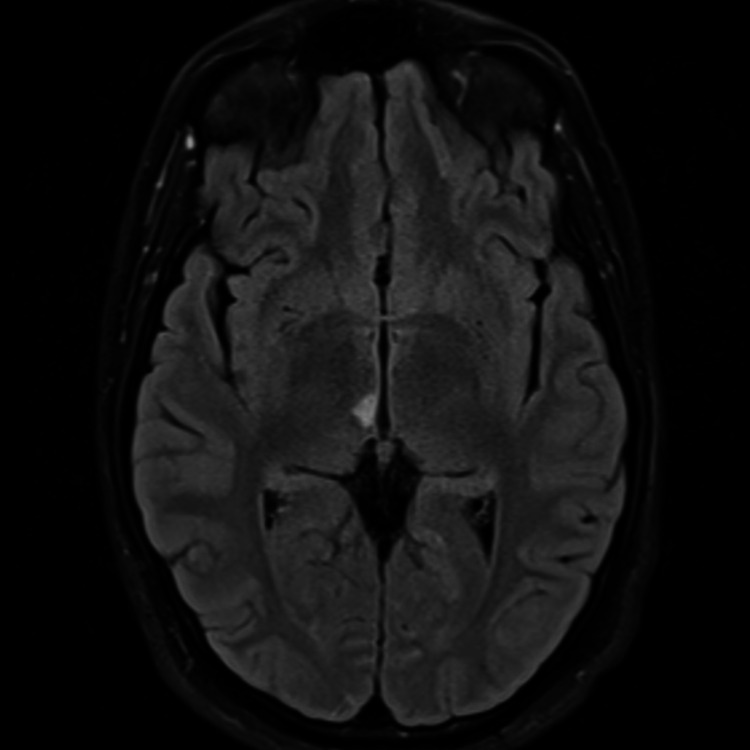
MRI of the brain with T2 weighted image shows small infarctions at the right thalamus.
